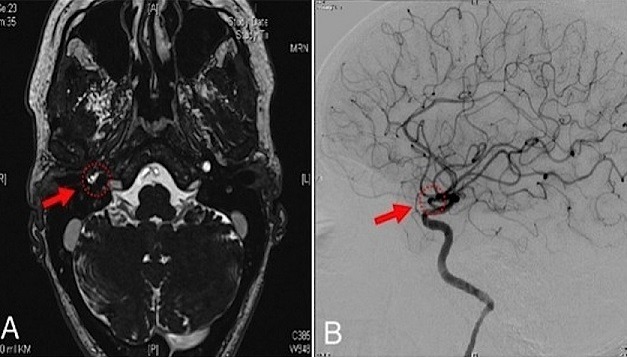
MRI (A) and angiography; (B) showing an aberrant internal carotid artery
